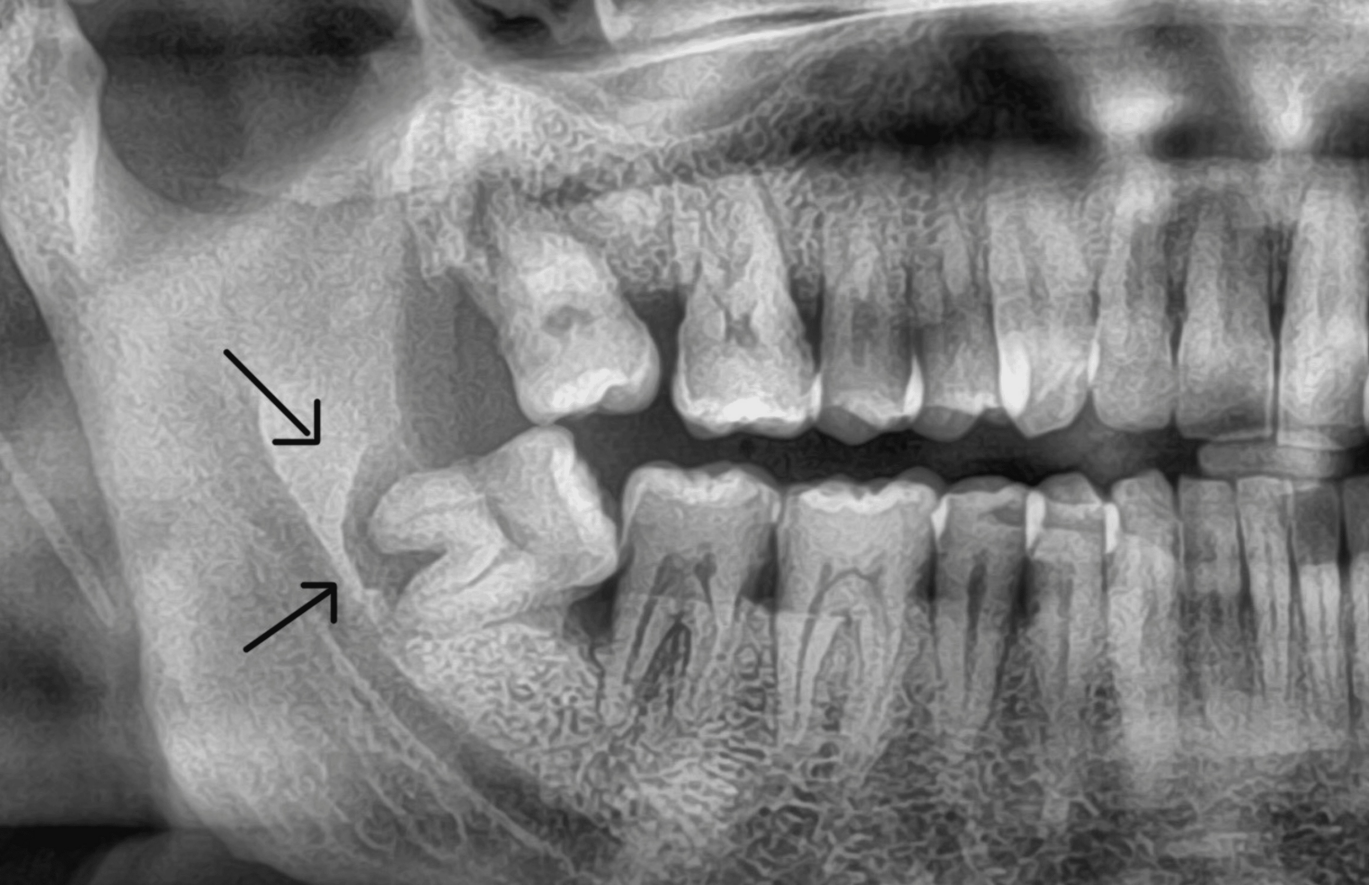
Radiograph showing well-defined radiolucency involving the distal root of mesioangular impacted 48. There is bone loss related to the distal aspect of 47 and the mesial aspect of 48.
